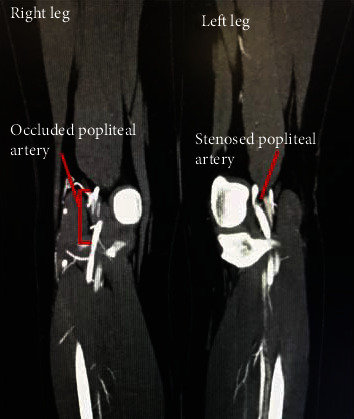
Computed tomography angiography demonstrating a coronal view of the behind knee popliteal arteries, with labelled total occlusion of the right popliteal artery and tight stenosis of the left popliteal artery.
